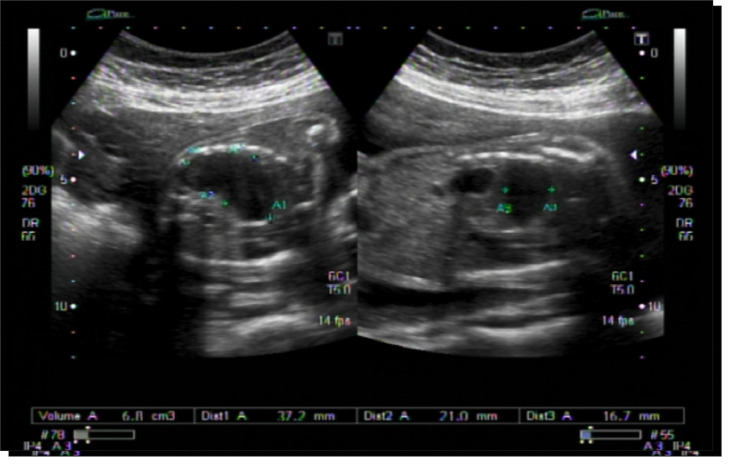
Fet al ultrasound showing the intrathoracic cystic lesion.
